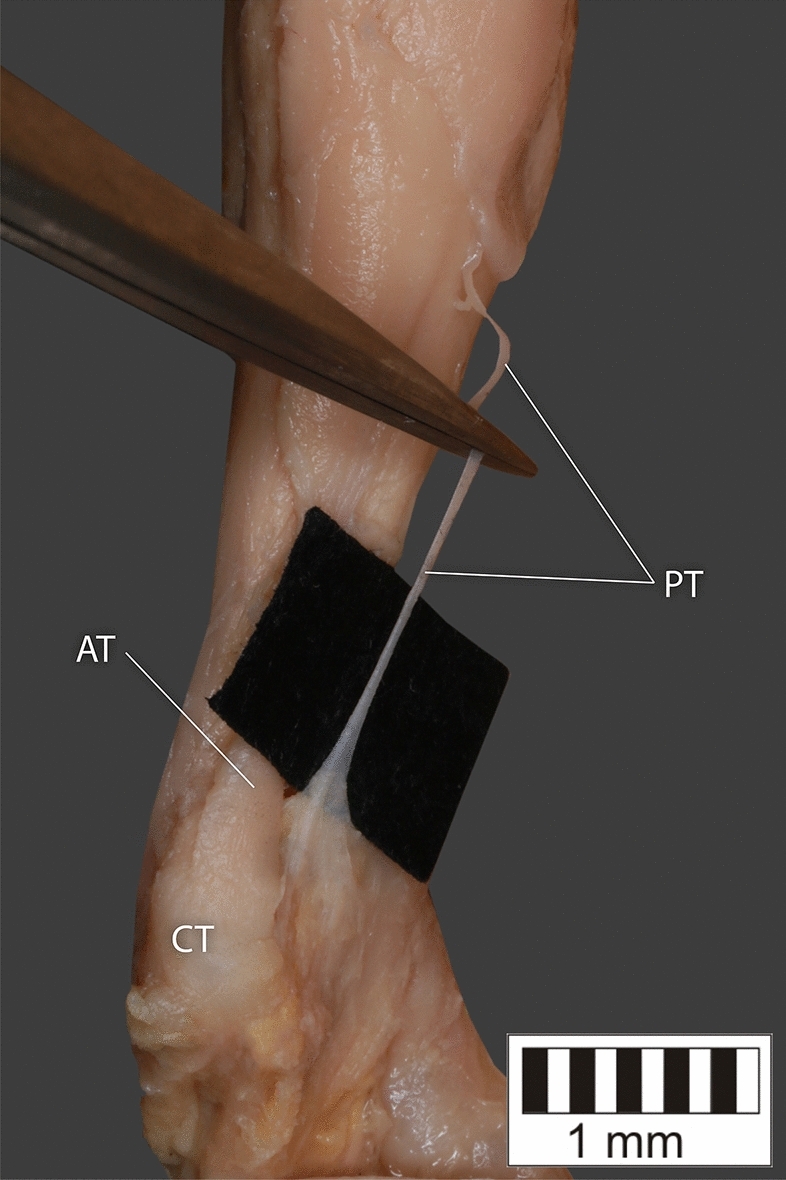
Type VI of plantaris tendon. *CT* calcaneal tuberostity, *AT* Achilles tendon, *PT* plantaris tendon.
